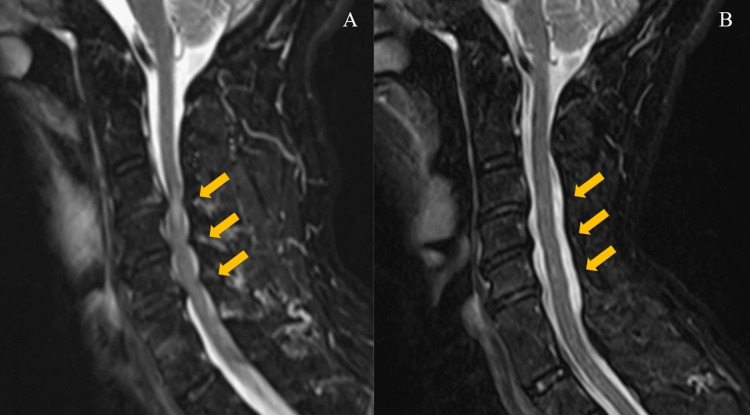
Cervical MRI with short T1 inversion recovery (STIR) sequence Cervical MRI before (A) and after (B) surgery. Decompression surgery (C3-6 laminoplasty (orange arrows) with C2 and C7 dome laminectomy) was performed about one month after admission.
